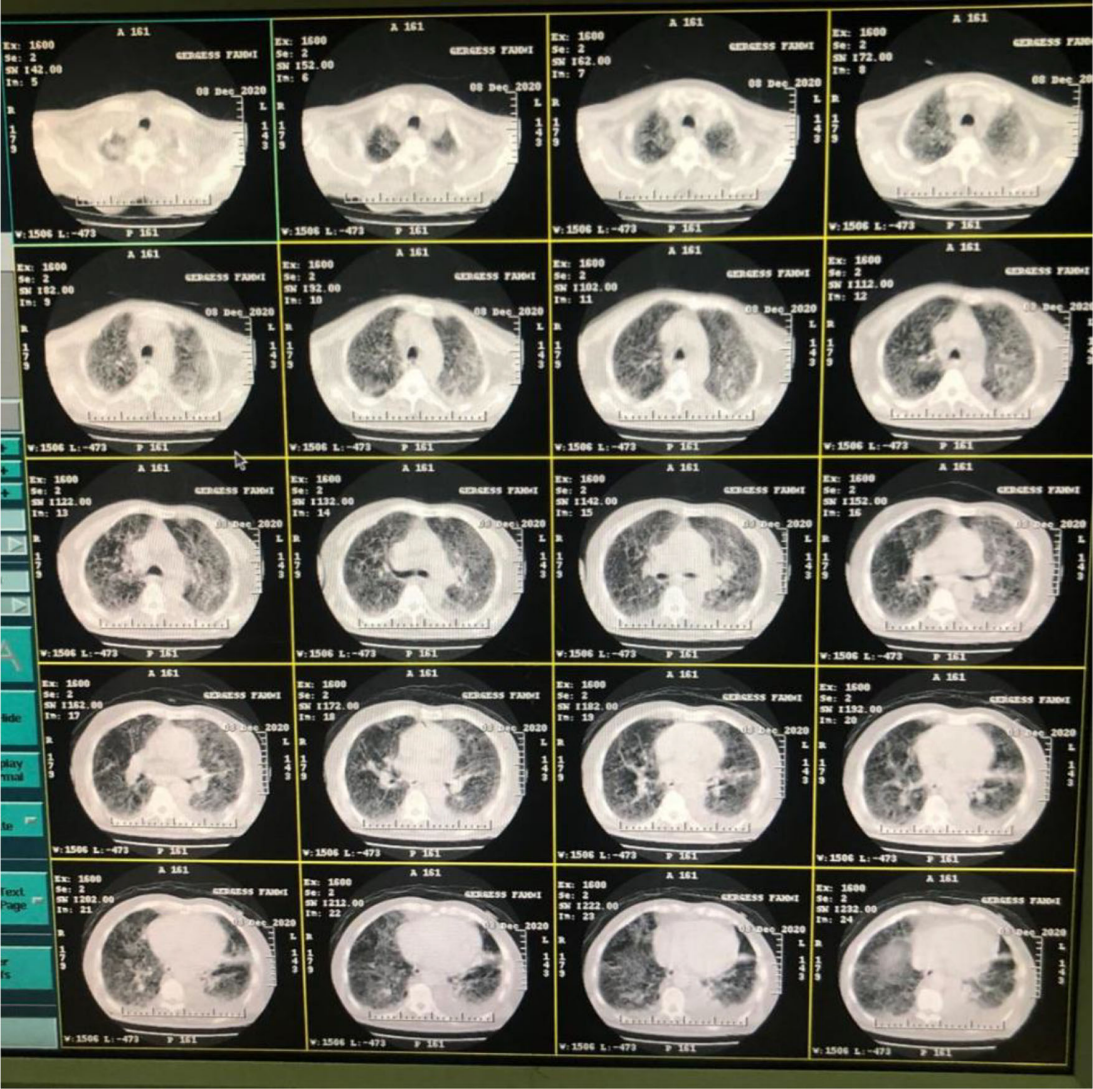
Chest computed tomography of case 1 on hospital arrival.
